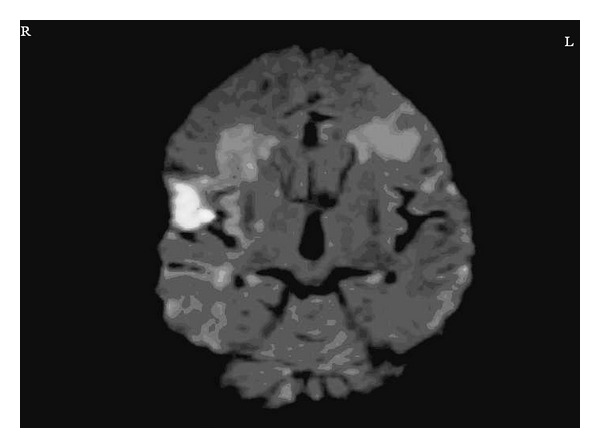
Diffusion-weighted magnetic resonance imaging demonstrates right frontal opercular restricted diffusion which is diagnosed as acute opercular infarct (R: Right, L: Left).
